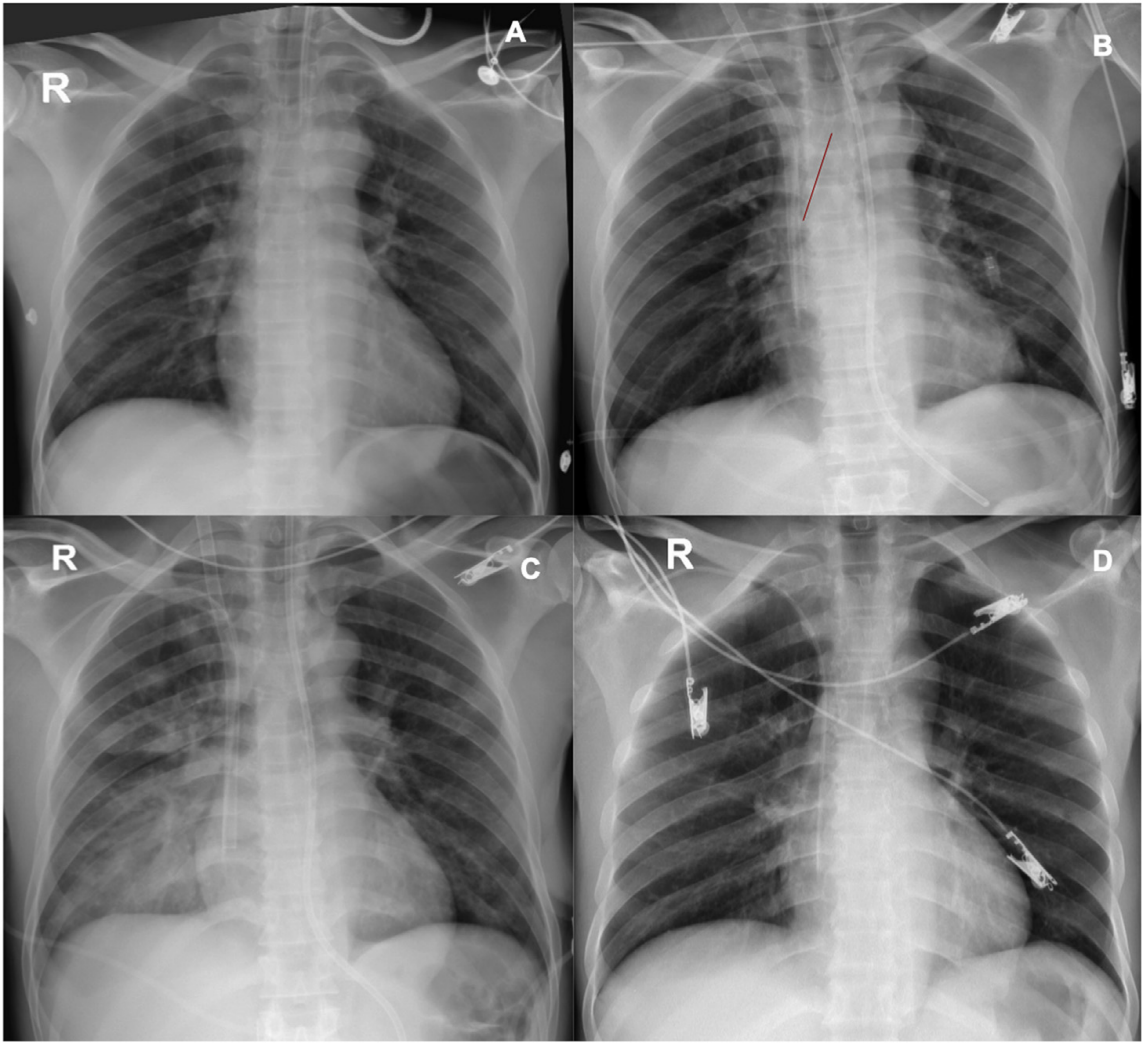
A. Anteroposterior chest X-ray taken at arrival where the orotracheal tube is in position, no pulmonary infiltrates, nor pleural effusion. B. Chest x-ray showing the jugular vein cannula for VV ECMO. The red line shows what was retrospectively identified as a possible foreign body in the airway. C. Chest x-ray with right lower lobe pulmonary alveolar infiltrates D. Chest x-ray at discharge without infiltrates or other alterations.
